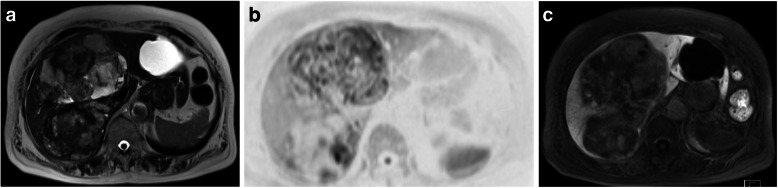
Magnetic resonance imaging findings. a Magnetic resonance image showing a tumor with mixed high- and low-intensity signals on T2-weighted imaging (HASTE). b Diffusion-weighted image showing a high signal intensity of the tumor. c The dynamic 15-mm hepatobiliary phase image of the prolongation of the internal contrast effect
